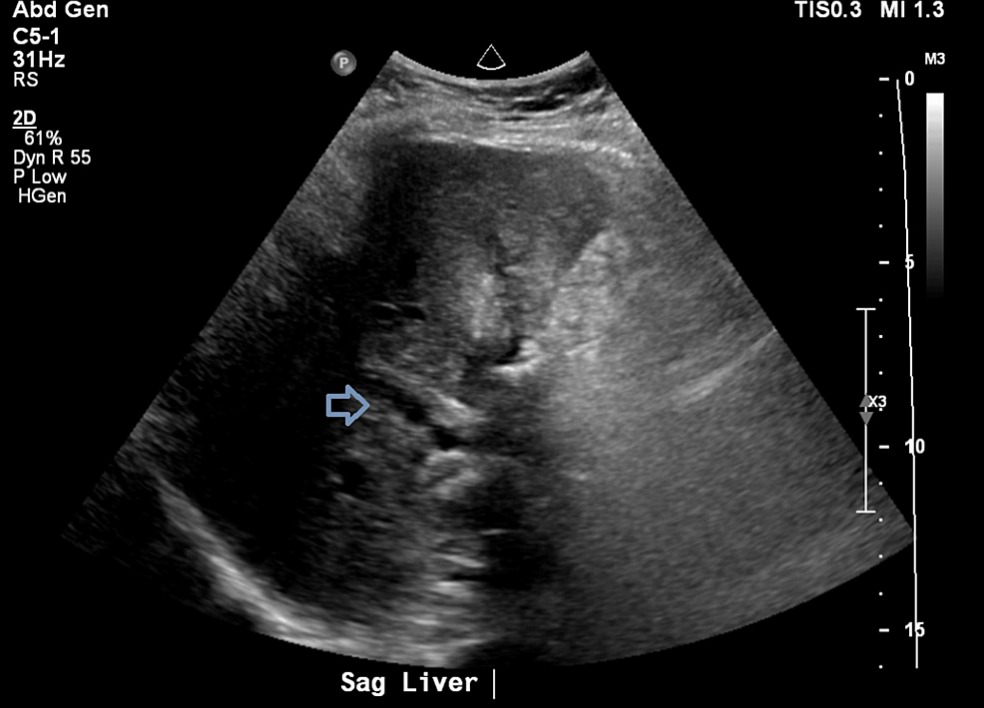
Ultrasound imaging on return admission showing biliary ductal dilation.
